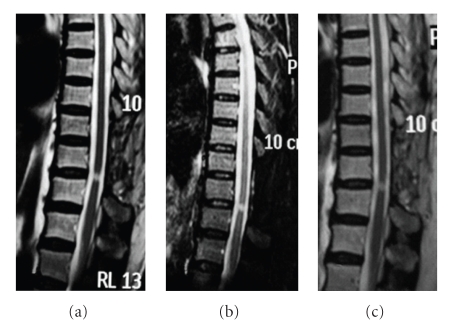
A 67-year-old female suffered from progressive paraparesis and paresthesias for 1 month. There is a small demyelinating lesion at the level between T9 and T10. Steroid pulsed treatment worsened her condition. After ATT, her weakness quickly improved. She tried to stop ATT two times during the early stage of treatment due to drug side-effects, but failed because her condition grew worse. Although she got better, the lesion seems to have not changed after 18 month treatment. (a) Sagittal T2-weighted image reveals a small high intensity signal between T9 and T10 before our regimen; (b) sagittal T2-weighted image after 6 months of ATT; (c) the lesion still exists on sagittal T2-weighted images after 18 months of treatment.
